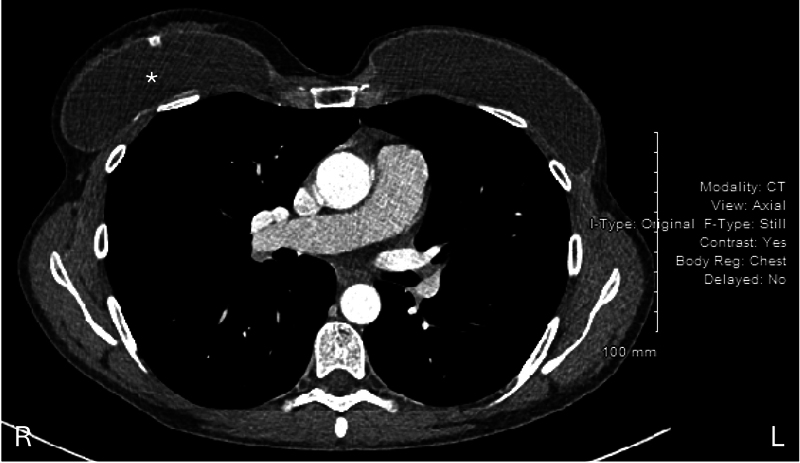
CT imaging demonstrating the right prosthesis (*) in the original position.
